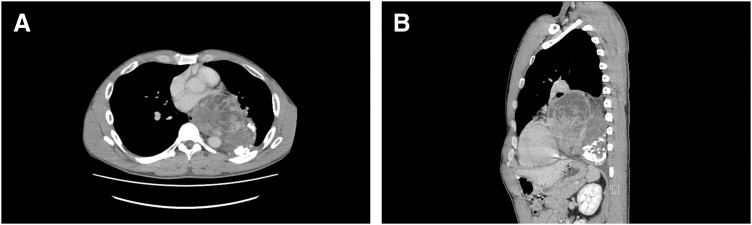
Chest CT findings of the patient. Chest CT scan showed a well-defined giant mass is observed extending from the middle to posterior mediastinum on the left side. The lesion showed heterogeneous enhancement with areas of hyalinization and calcification. (A) axial view. (B) Sagittal view.
